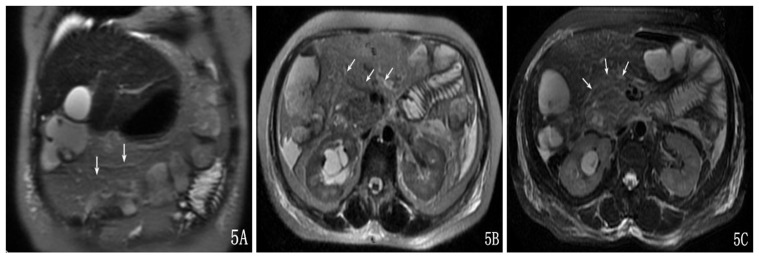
A 56-year-old female with moderate AP and TMI was Grade II. The transverse mesocolon showed edema and thickening on a coronal SSFSE T2-weighted image (A), on an axial SSFSE T2-weighted image (B) and on an axial SSFSE T2-weighted image with fat suppression (C).
